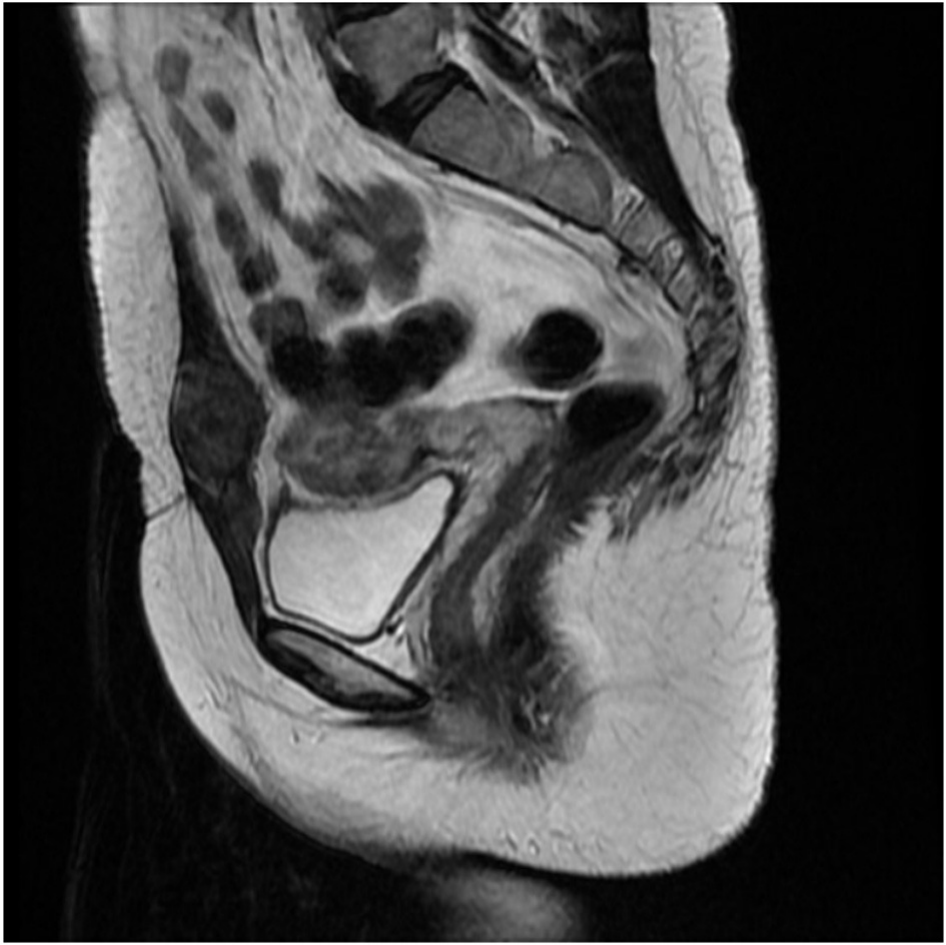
Magnetic resonance imaging (MRI) revealed a 3 × 2.6-cm soft tissue mass in the anterior abdominal wall.
